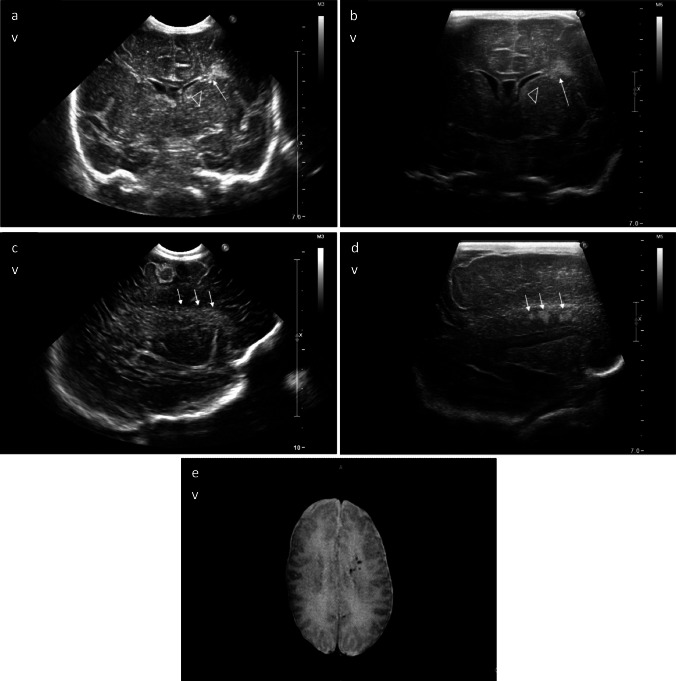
Intraventricular haemorrhage (IVH) complicated by periventricular haemorrhagic infarction (PVHI) in a preterm infant (30-week gestation, 11 days of life). a, b Coronal scans. The PVHI is presented by an echogenic lesion (white arrow) in the left periventricular white matter, ipsilateral to the IVH (arrowhead). Note that the PVHI is an area of increased echogenicity that is asymmetric, wedge-shaped, and located above the anterior horn of the lateral ventricle. In preterm infants, these lesions should be distinguished from normal periventricular echodensities that are bilateral, symmetric, round-shaped, and located laterally to the anterior horns of the lateral ventricles (as shown in Fig. S1, a). c, d Left parasagittal scans. The micro-convex transducer (c) shows subtle hyperechoic lesions in the left periventricular white matter (arrows): however, this scan displays diffuse echogenicity with blurred margins, which can be confused with the typical periventricular hyperechogenic halo of prematurity. By contrast, with the very high-frequency probe (d), the PVHI appears inhomogeneous (arrows) with focal hyperechoic areas in contrast with physiological hyperechogenicities (Fig. S1, g). e MRI at term equivalent age. T2 gradient echo image (axial plane) at the level of the centrum semiovale shows small areas of venous infarctions in the left periventricular white matter, confirming the diagnosis of PVHI.
